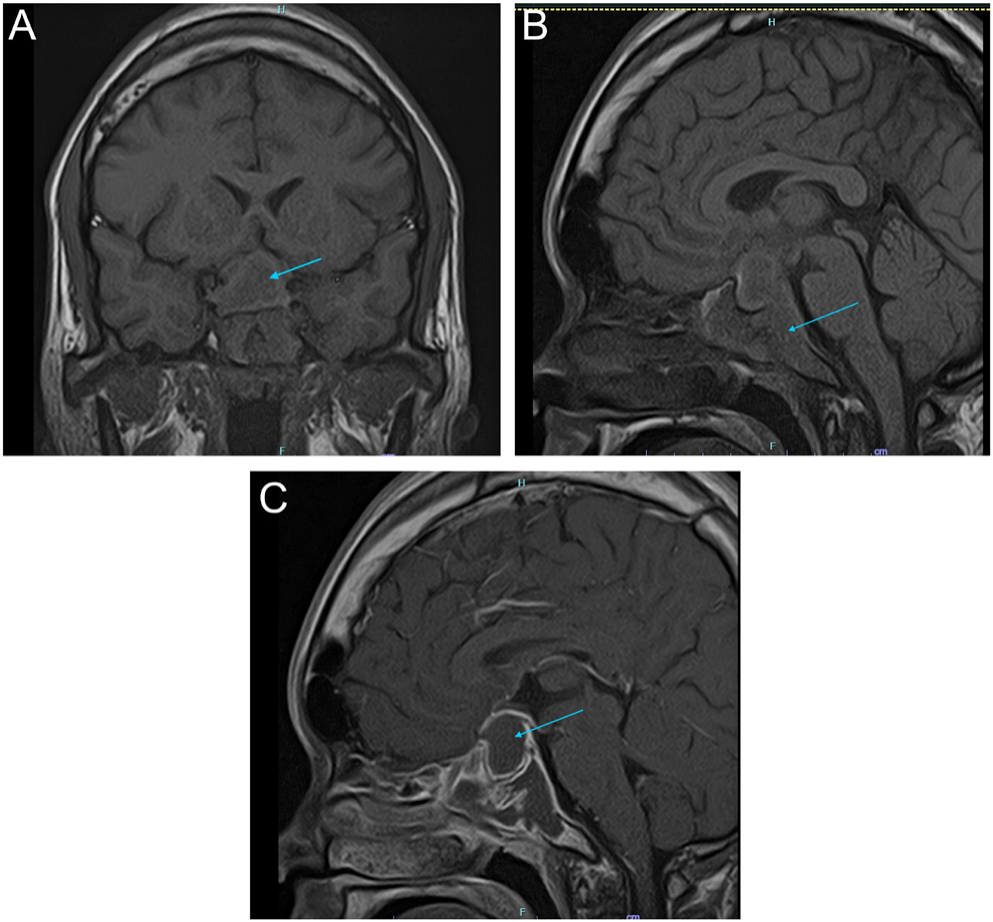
(A) T1-weighted coronal image pre-contrast – isointense sellar mass with erosion into bilateral cavernous sinus. (B) T1-weighted sagittal image pre-contrast – isointense sellar mass with erosion into sphenoid sinus and clivus. (C) T1-weighted sagittal image – peripheral enhancement seen post-contrast.
